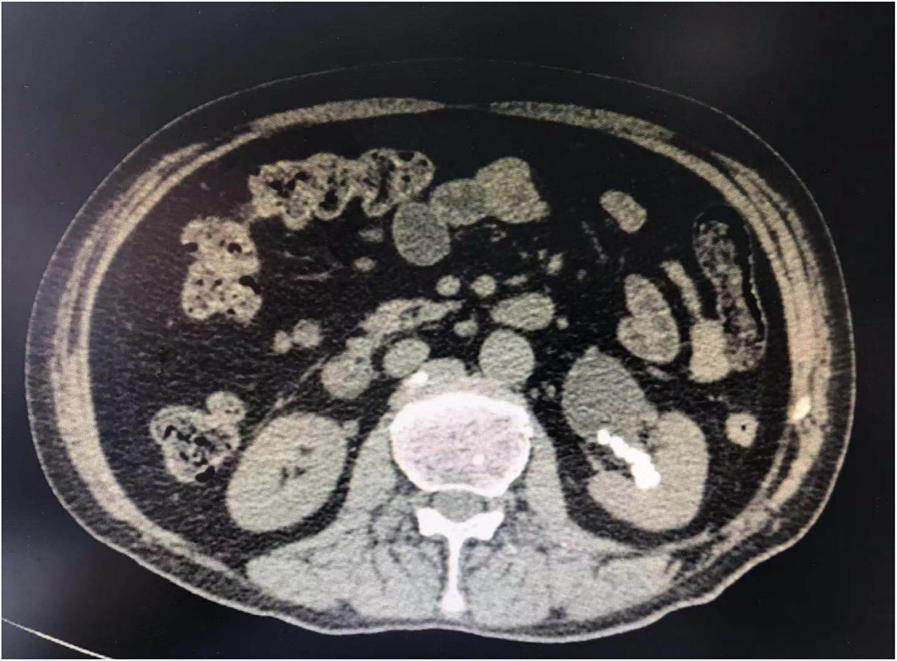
CT scan showed left renal calculi and hydronephrosis
